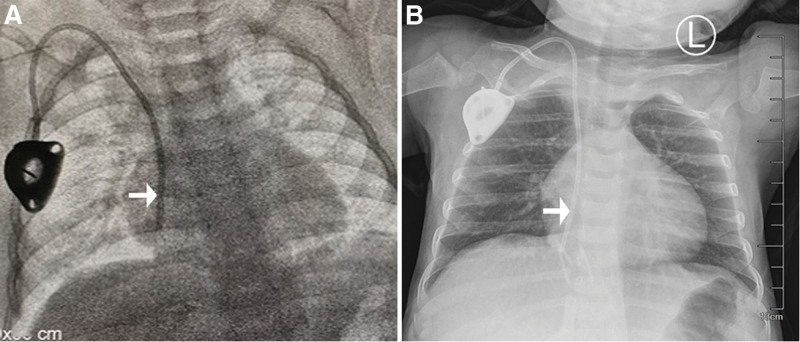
Intraoperative and postoperative images of the first catheterization. (A) Fluoroscopy shows the tip of the catheter (white arrow) in the right atrium. (B) Chest roentgenogram shows the tip of the catheter (white arrow) in the right atrium.
